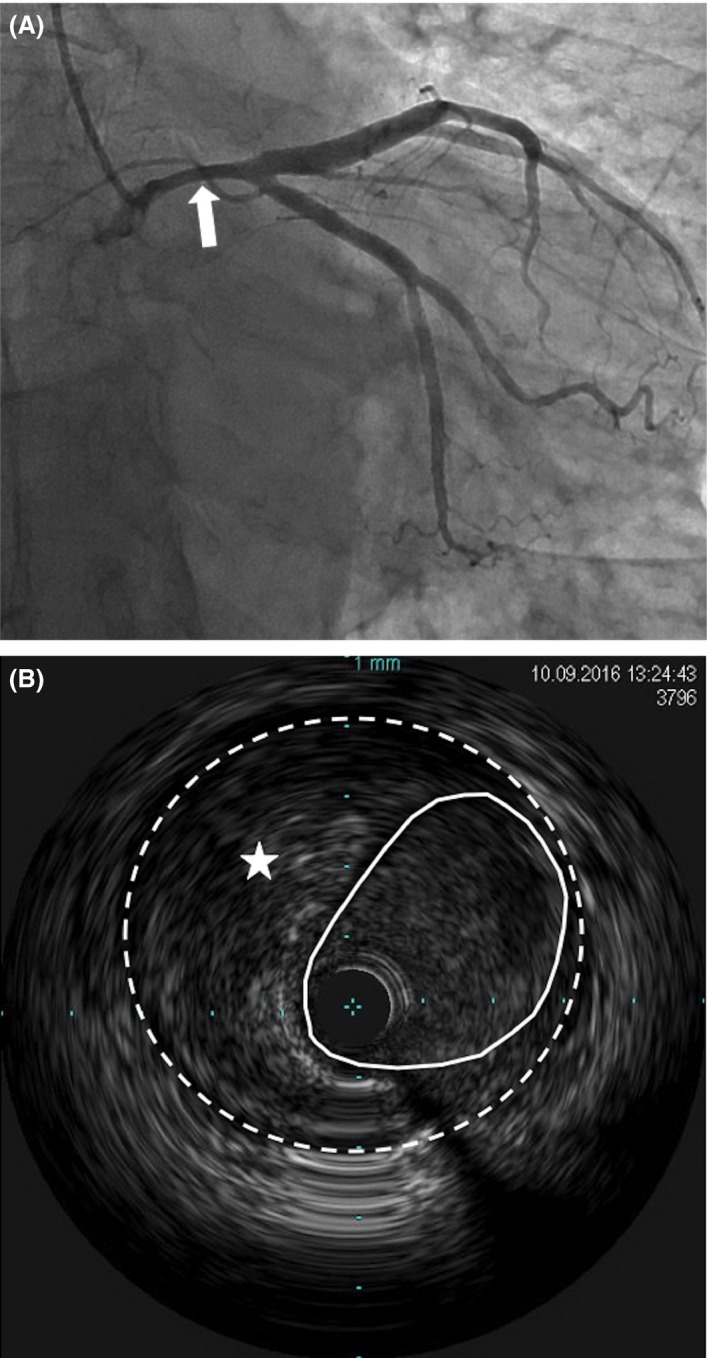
Initial cardiac catheterization and intravascular ultrasound. (A) Caudal view of the left main coronary artery with diffuse loss of vessel diameter (arrow). (B) Left Main intravascular ultrasound exhibiting intramural hematoma (star) with luminal narrowing (dotted line). The solid line represents the adventitia. No obvious dissection flap was identified.
